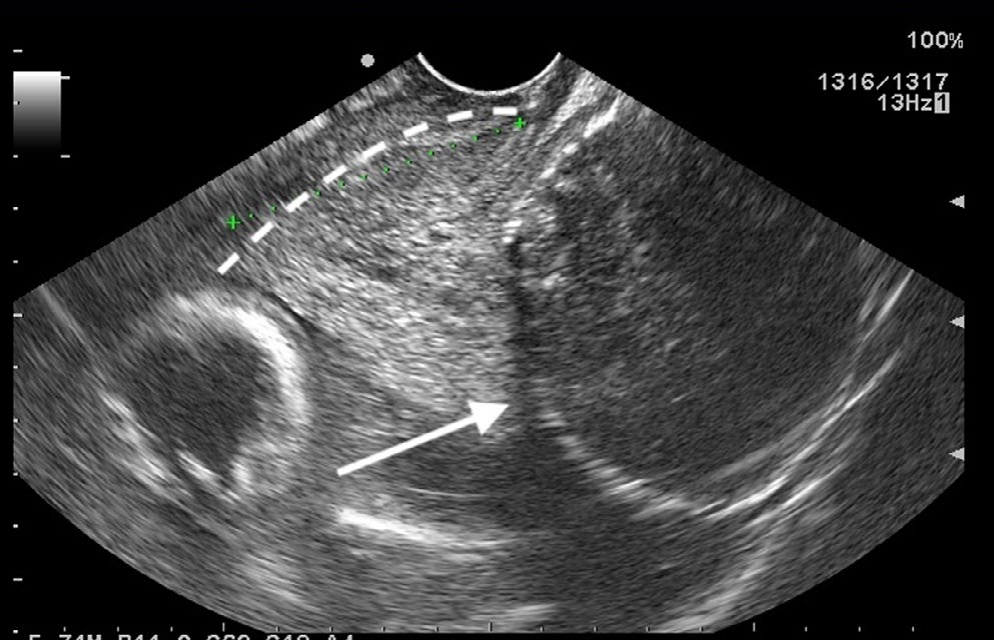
Transvaginal ultrasonography at 17 wk of gestation. The cervix (dotted white line) is displaced forward, and an 8‐cm uterine fibroid (white arrow) is visible in Douglas' pouch
